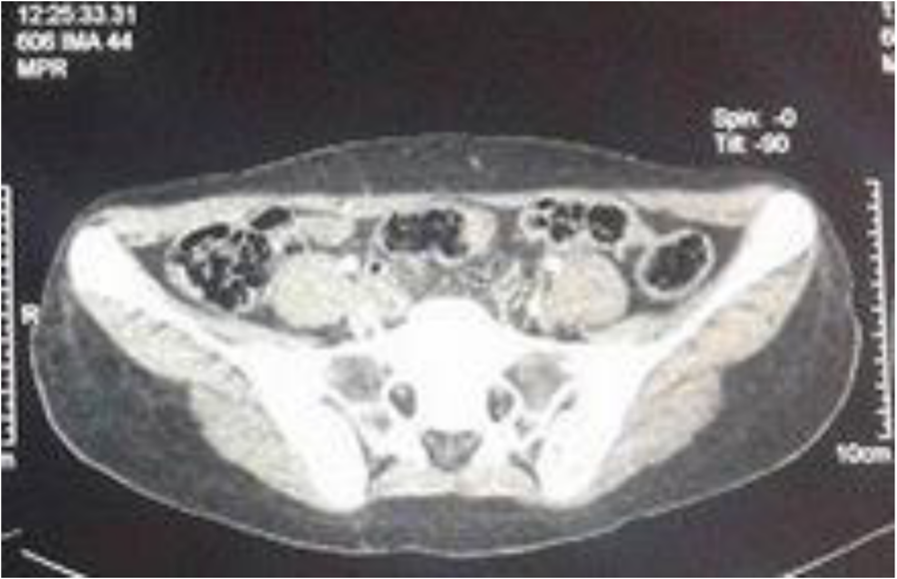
Post-chemotherapy axial computed tomography showing a complete response in lymph nodes
